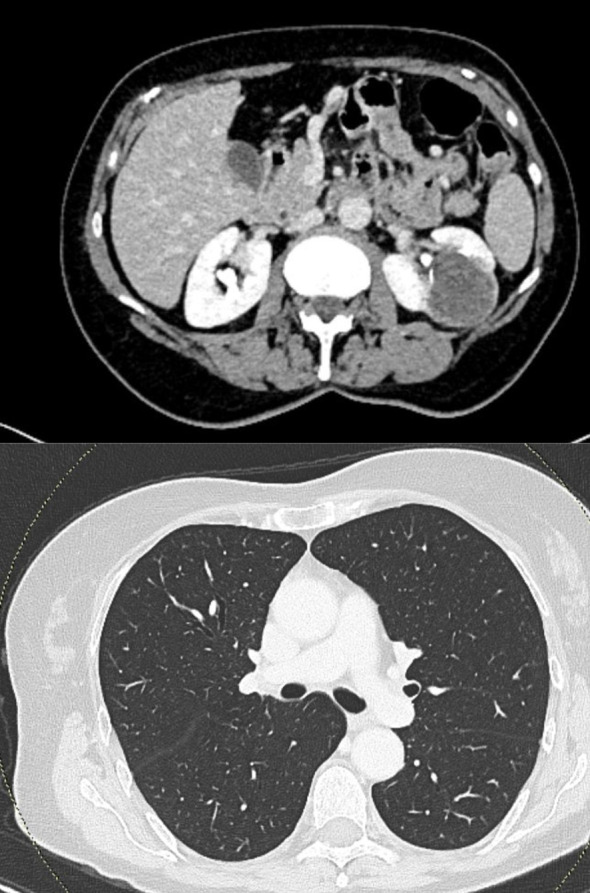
CT scan after four cycles of therapy showing reduction of the left renal lesion to 37 × 35 mm (top), disappearance of the previously noted paracolic nodules (top), and reduction of bilateral pulmonary nodules (bottom).
